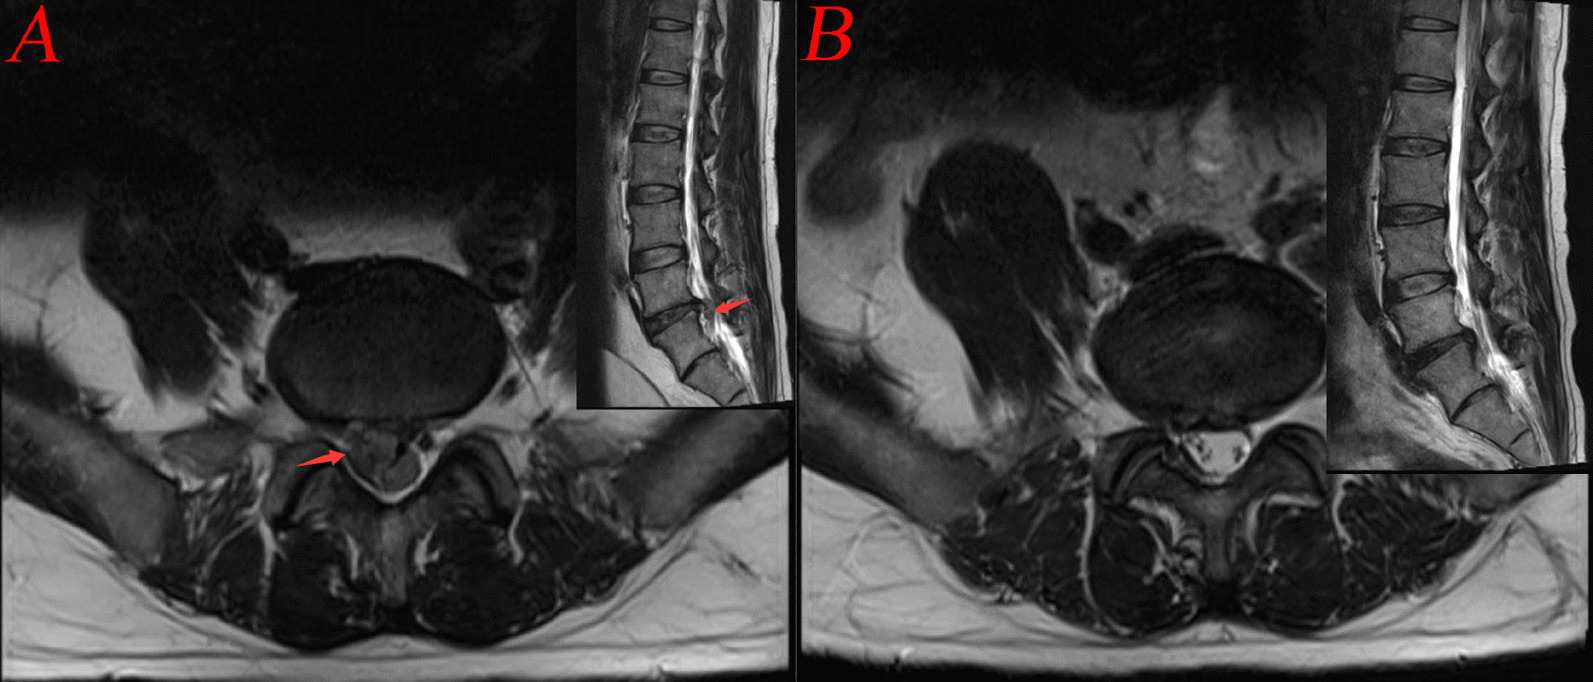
A Preoperative MRI (arrow: downmigrated disc herniation); B Postoperative MRI
